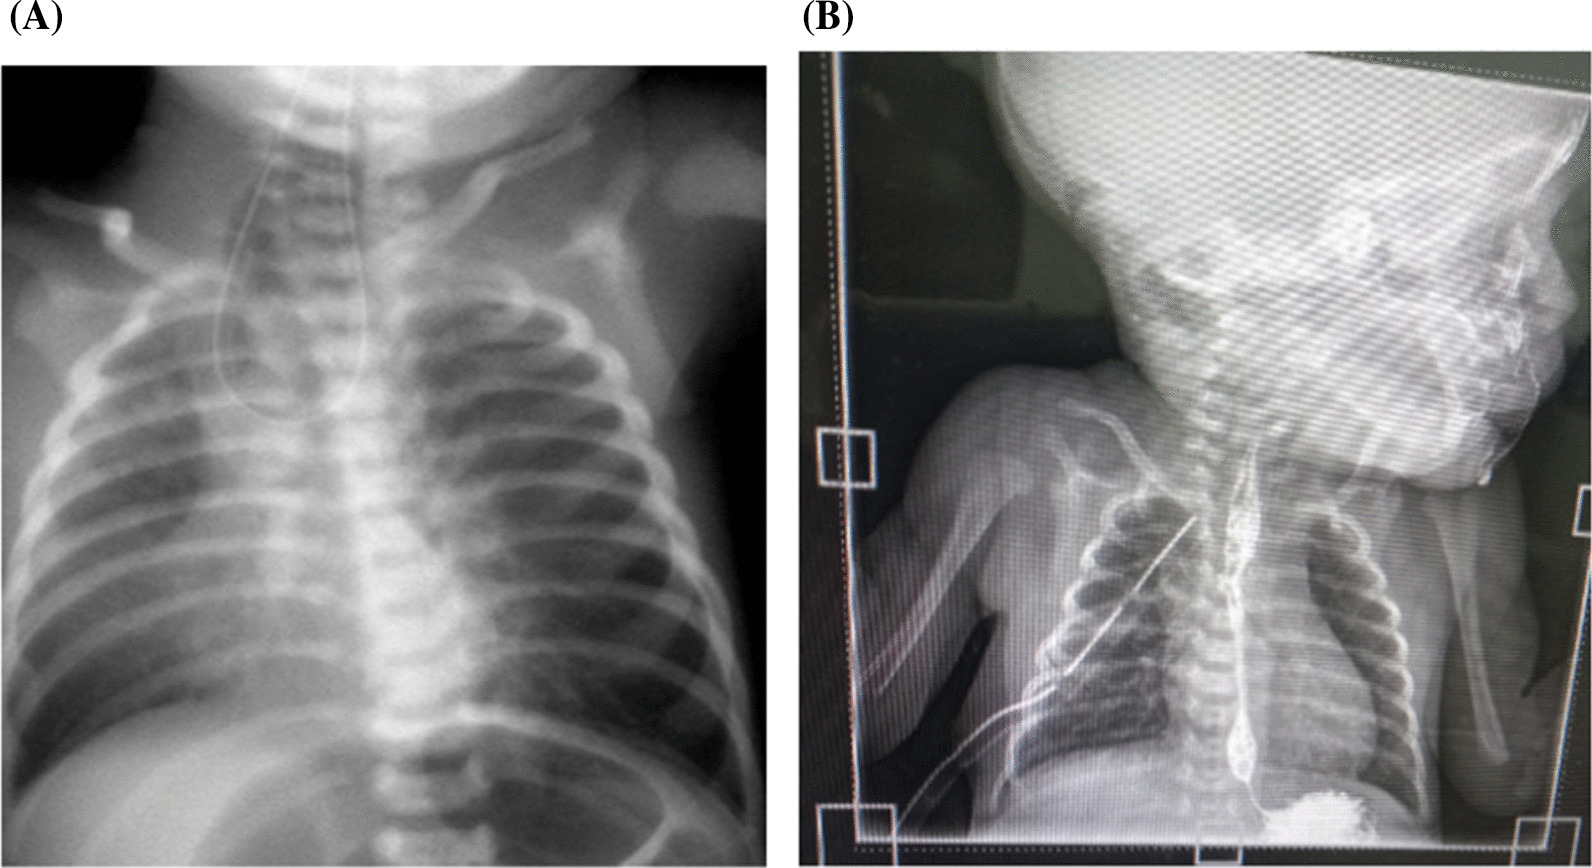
A Chest X-ray showing coiling of nasogastric tube. B Chest X-ray showing healed anastomosis
